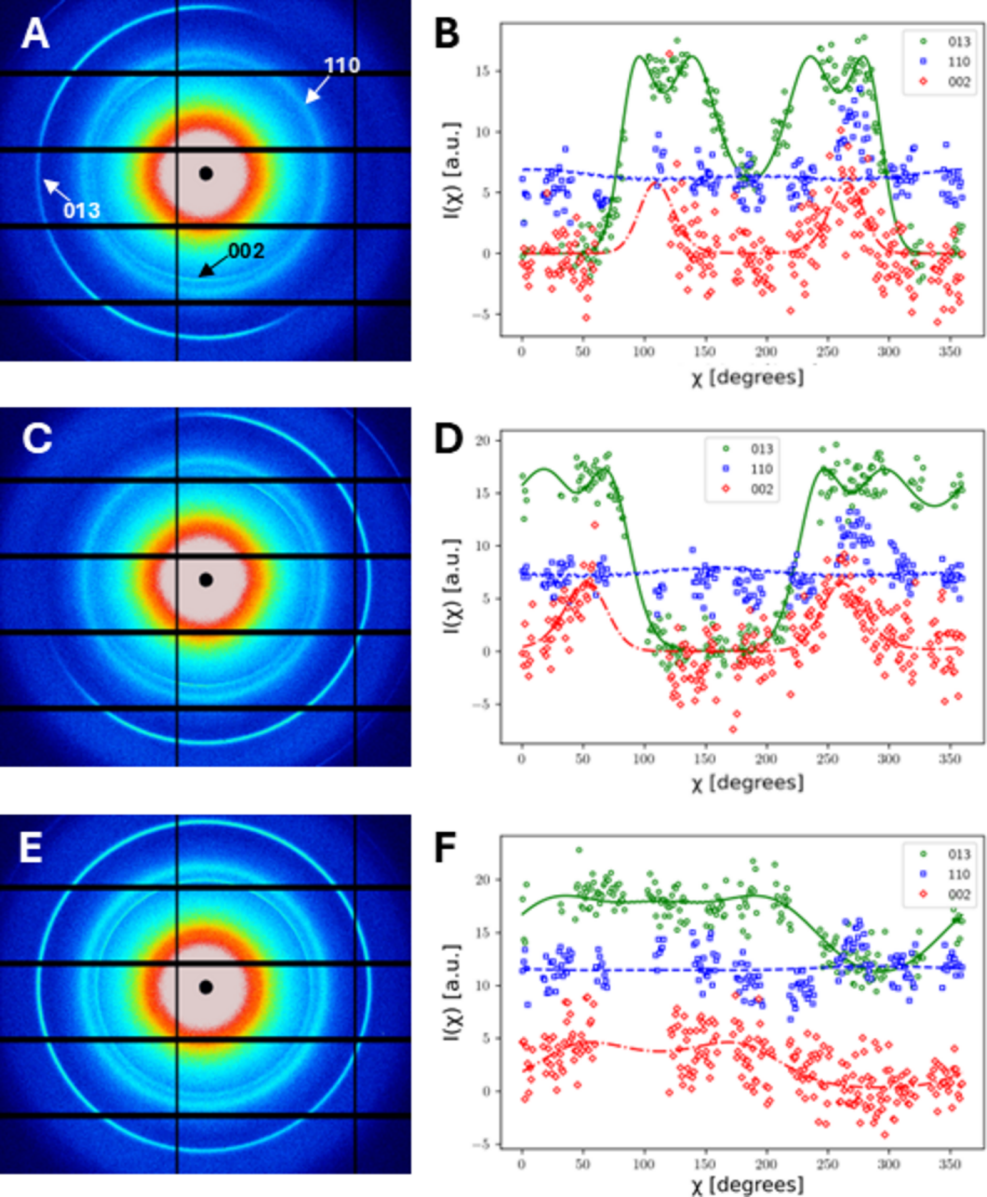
Experimental data and model fits from cuticle samples in the L1 geometry. (Left) Experimentally recorded X-ray patterns from three samples. The (002), (110) and (013) reflections from chitin fibres are indicated by arrows in panel (*a*). (Right) Azimuthal intensity profiles *I*(χ) of the three reflections. Panels (*a*) and (*b*) show data for sample S01, panels (*c*) and (*d*) for sample S02 and panels (*e*) and (*f*) for sample S03. The colour coding is as follows: axial reflection 002, red; equatorial reflection 110, blue; 013, example of the general case, green. Open circles, squares and diamonds are experimental data and solid lines are the model fits.
